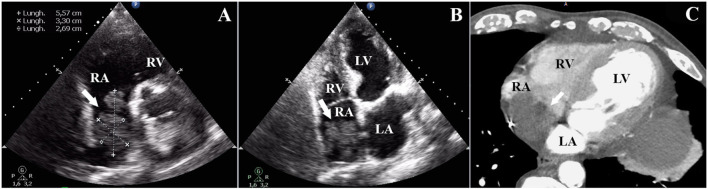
(A,B) Modified short-axis view and four-chamber view showing irregular thrombotic mass pointing toward the tricuspid valve, into the right ventricle. (C) Venous phase, contrast CT scan with evidence of intraluminal hypo-dense filling defect, with irregular profile, nearly occluding an enlarged right atrium.
